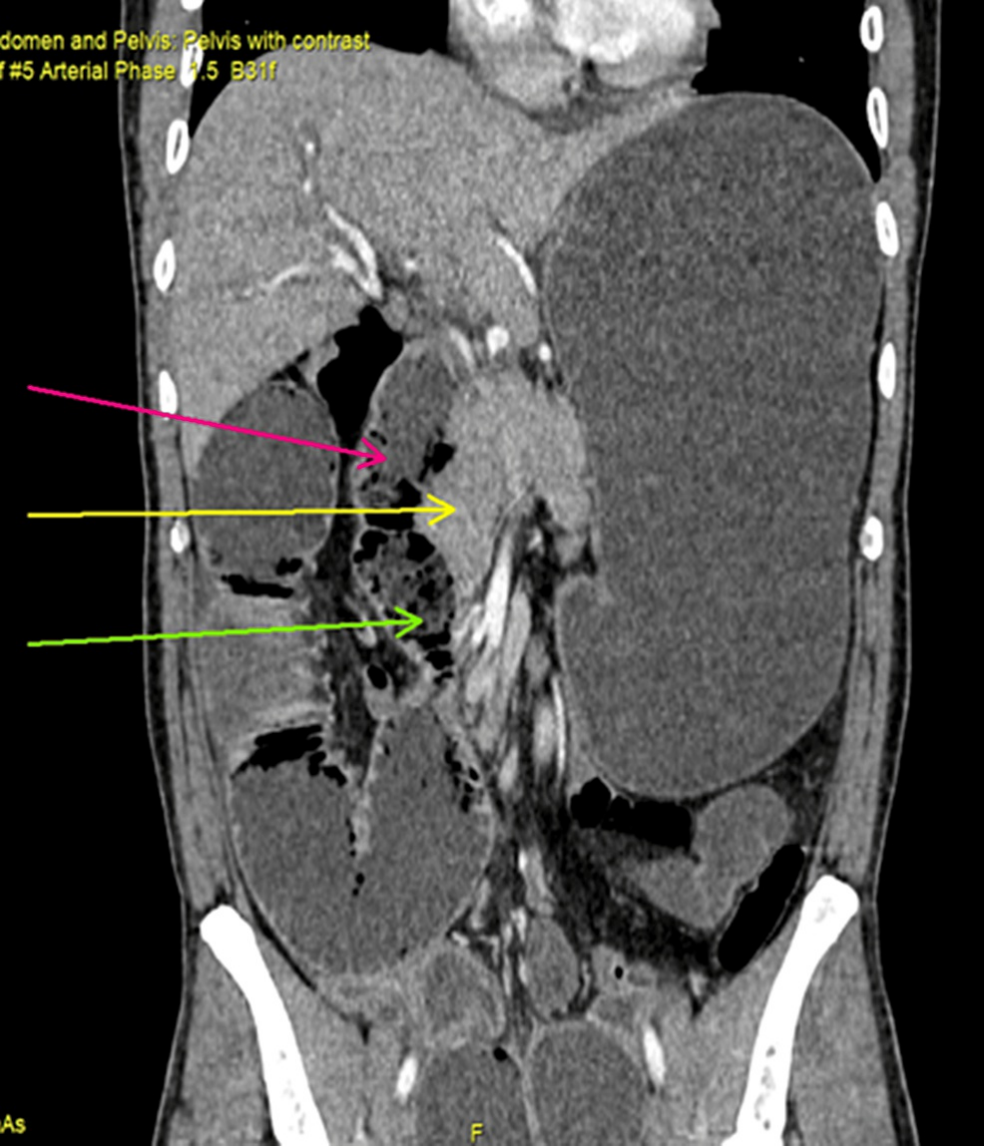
Coronal view of venous phase of contrast enhanced CT scan of abdomen and pelvis showing second part duodenum (pink arrow), uncinate process of pancreas (yellow arrow), duodenojejunal flexure of the right of abdomen (green arrow)
